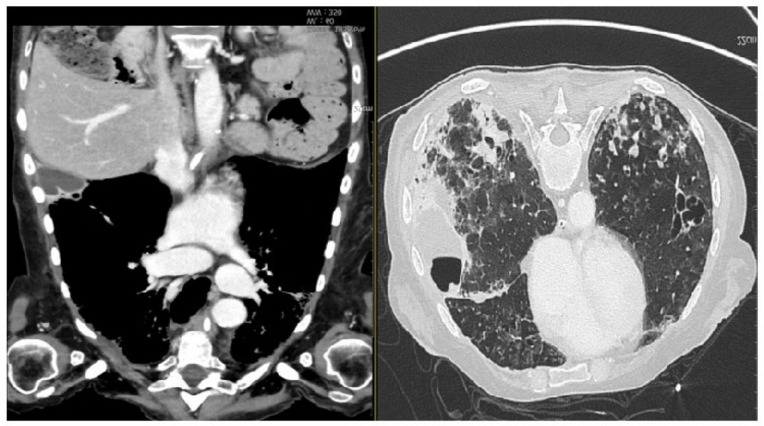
CT scan showing (during hospitalization) pneumonia by Cytomegalovirus. At the lateral-basal segment of the right inferior lobe, in the subpleural level, the gross intraparenchymal collection with hydro-aerial content is compatible with the pneumatocele. In the lung window, multiple areas of parenchymal thickening are visible in the right basal location. (On the left, coronal section parenchymal window, on the right, axial section lung window). Partial laryngectomy (OPHL II B) with bilateral selective neck dissection (cervical nodal Robbins levels II–IV) and postoperative radiotherapy were planned to manage this case. Unfortunately, this planned surgical intervention was impossible because of the patient’s poor general conditions. Moreover, one of the follow-up CT scans of the chest (three months after the initial one) revealed the presence of bilateral diffuse multiple micronodules, which were considered early distant metastasis from the laryngeal adenoid cystic cancer (Figure 3).
